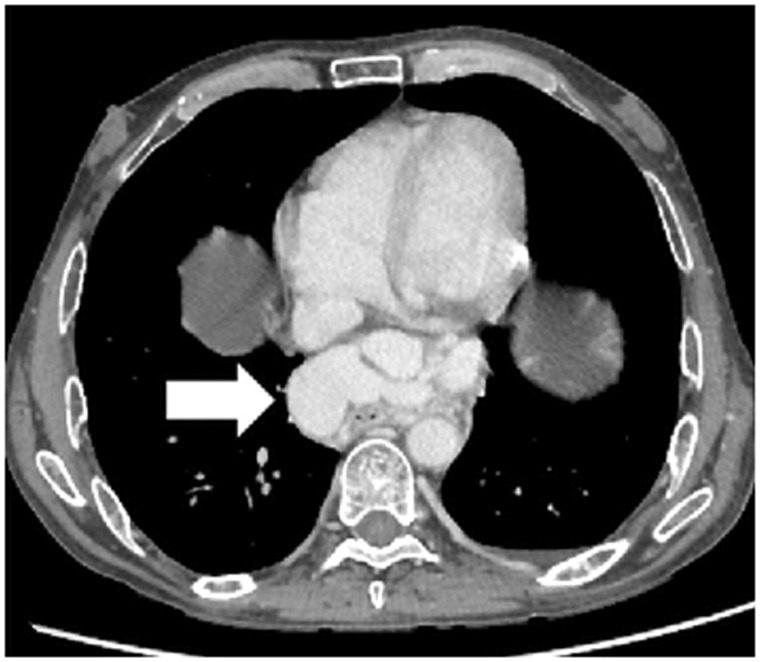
Intrathoracic portosystemic collaterals. Axial contrast-enhanced CT scan showing intrathoracic portosystemic collateral in the form of dilated paraesophageal veins (arrow) in a case of cirrhosis.
